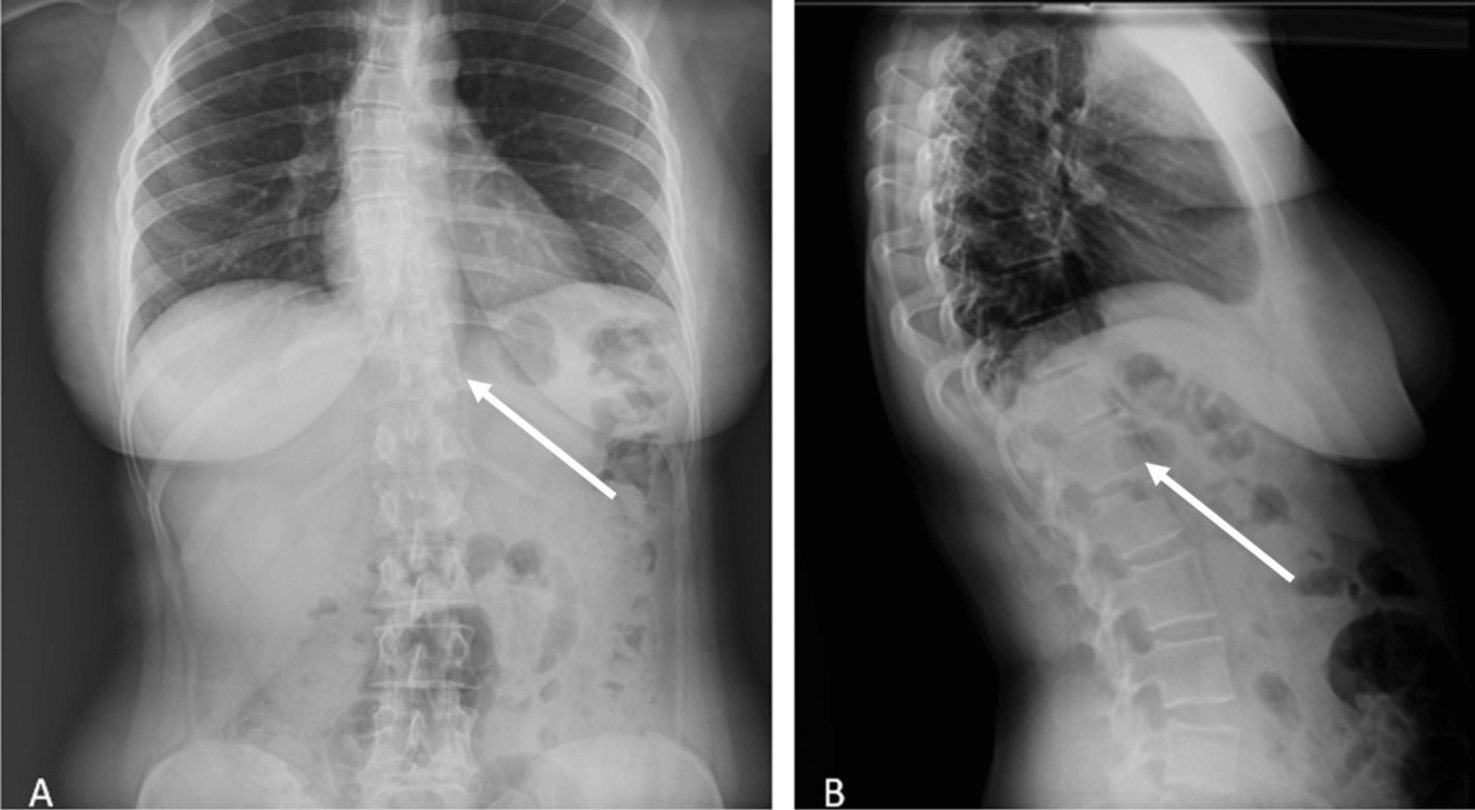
AP and lateral X-ray showing the lesion: (A) scoliosis AP X-ray images of the patient; (B) scoliosis lateral X-ray images of the patient. The white arrows indicate the lesion. AP: anteroposterior
